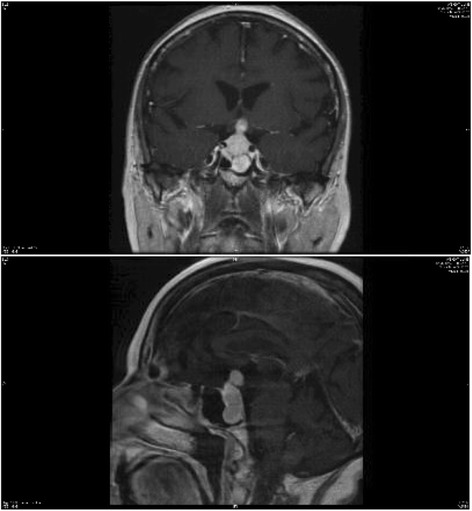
Sagittal and coronal MRI views, revealing a 34 × 12 × 15 mm intrasellar mass, extending into the sphenoid and cavernous sinuses
